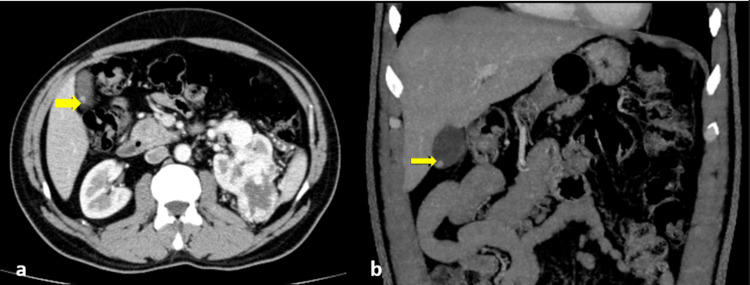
Contrast CT abdomen - axial (a) and coronal (b) images demonstrate a tiny enhancing intraluminal gall bladder lesion (yellow arrows) CT: computed tomography
